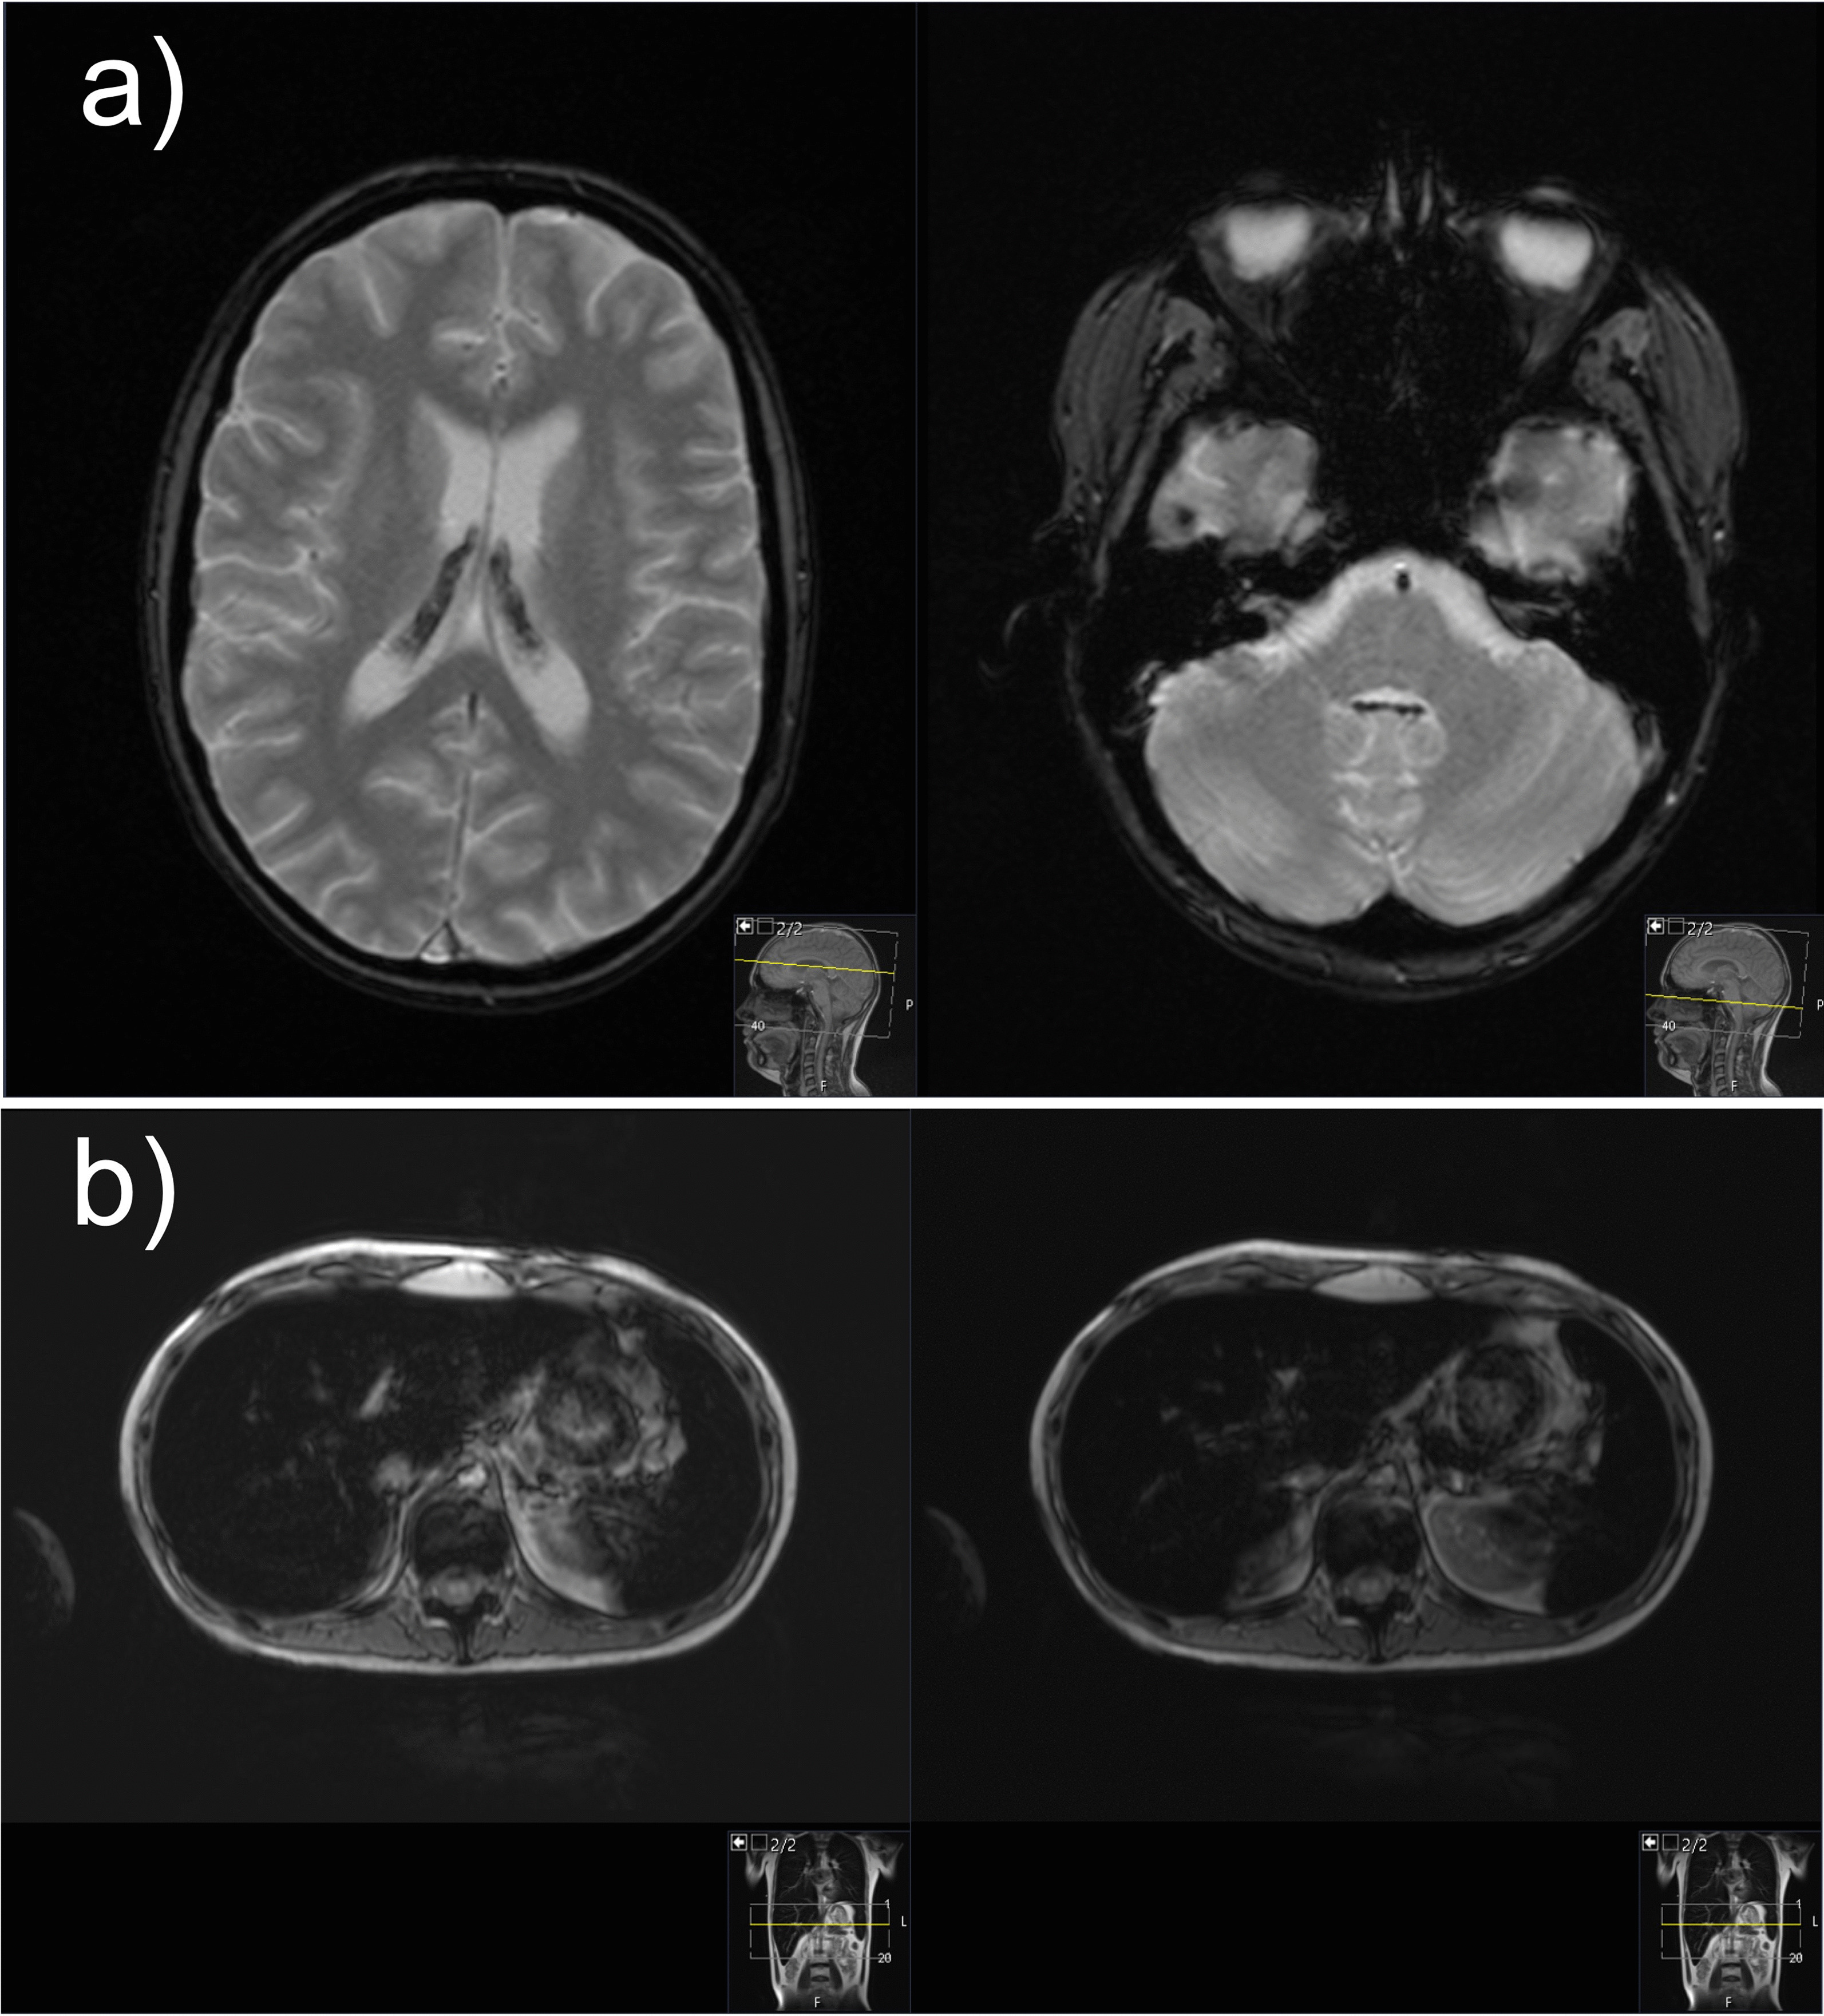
Magnetic resonance images of the brain and liver demonstrating iron overload. a Magnetic resonance imaging of the brain: axial T2-weighted gradient echo images demonstrate presence of hypointense hemosiderin deposits in the choroid plexus of both the lateral ventricles and fourth ventricle. b Magnetic resonance imaging of the liver: axial gradient echo sequences T2-weighted magnetic resonance image shows the liver hypointensity that is due to iron overload
